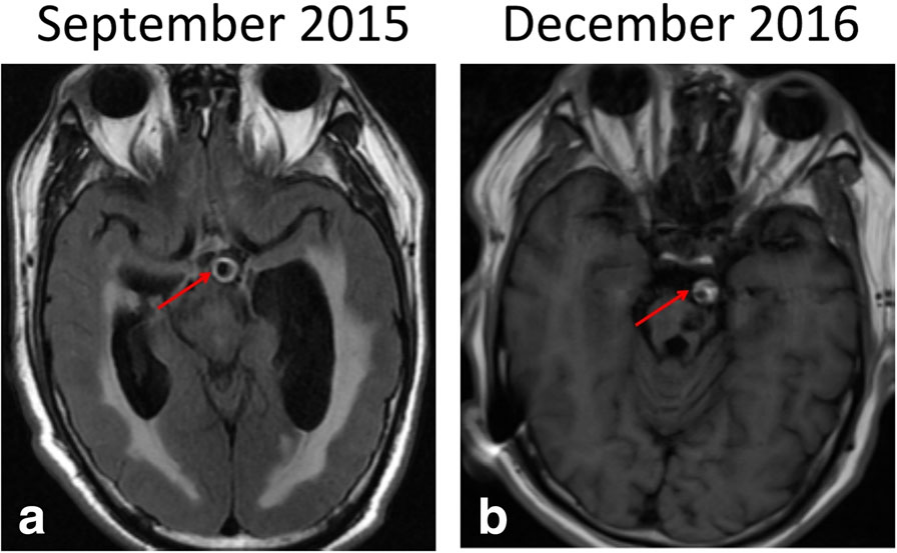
MRI-FLAIR scans. (a) A hyperintense rim near the vessel wall (FVH grade 2). (b) A hyperintense signal that nearly fills the entire BA lumen (FVH grade 3)
